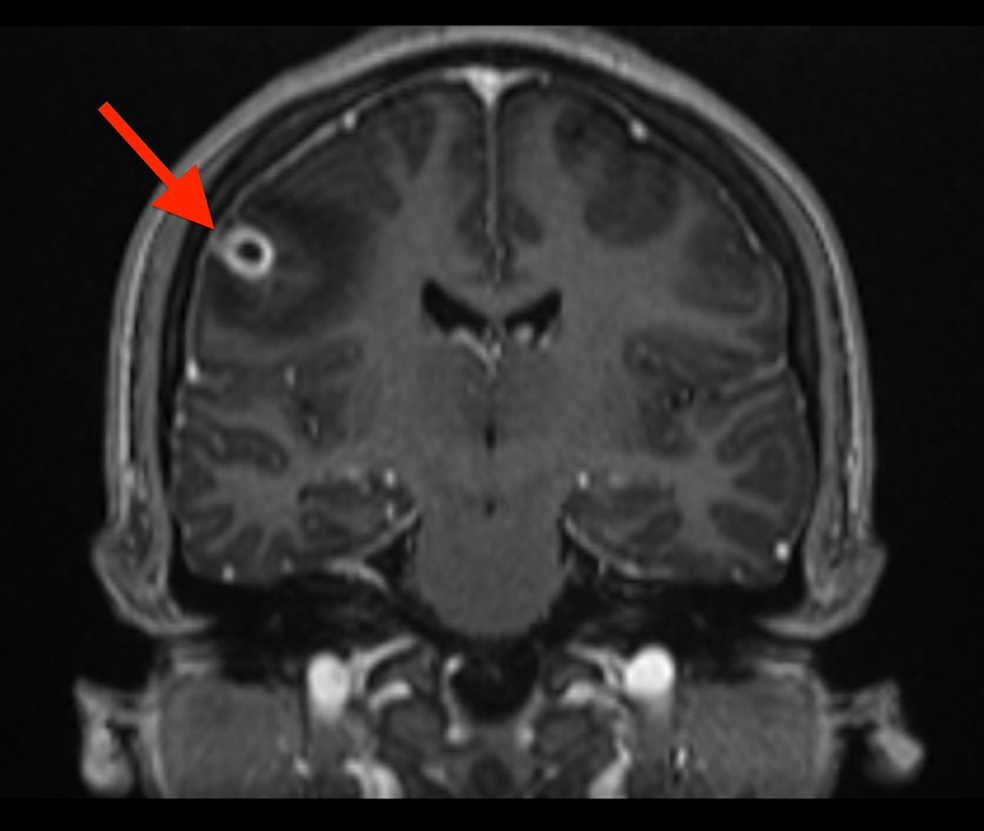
Coronal view MRI brain showing cystic mass with thickened peripheral enhancement (red arrow).
